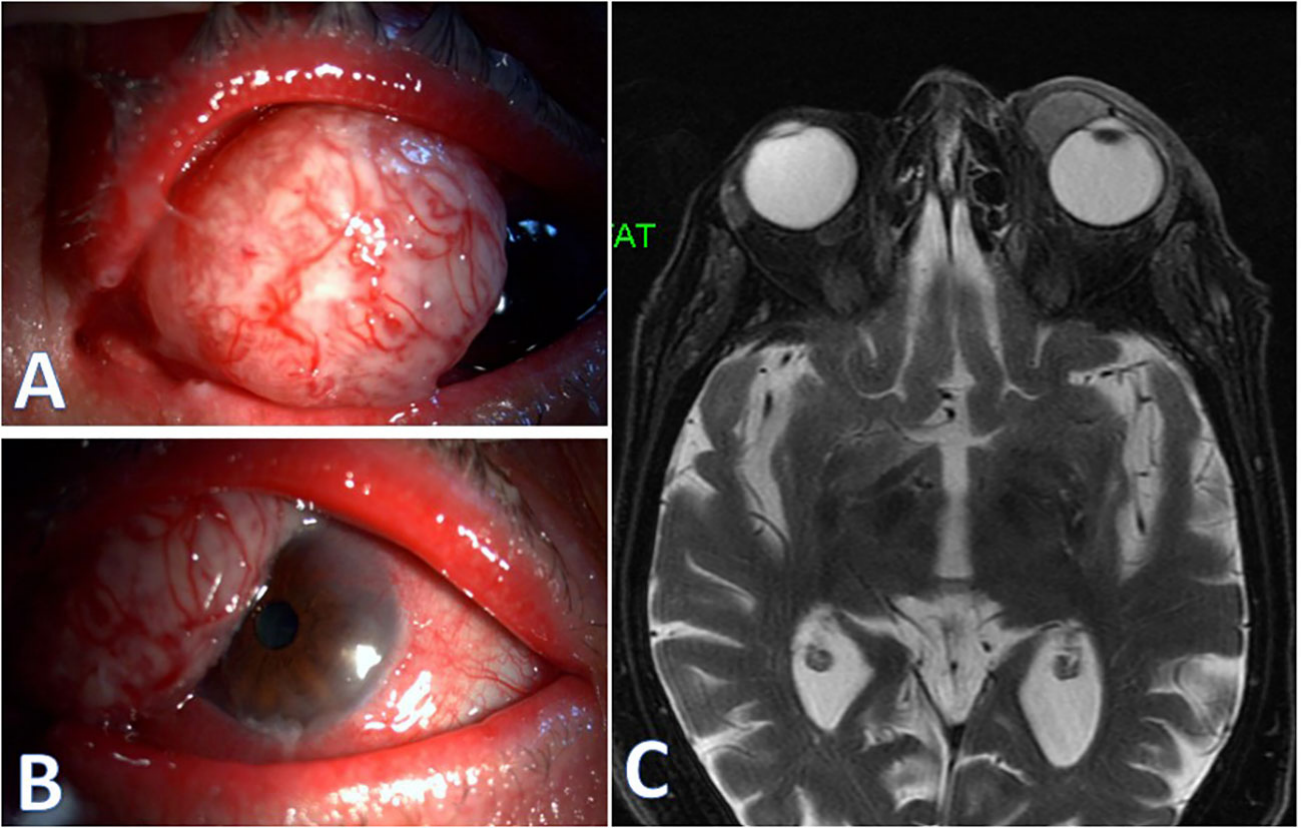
(A, B) Color slit-lamp photographs showing a large vascular mass covering approximately half of the corneal surface. (C) Orbital magnetic resonance imaging (MRI) with an arrow marking the lesion along the anterior medial globe wall, showing posterior extension toward the medial rectus region.
